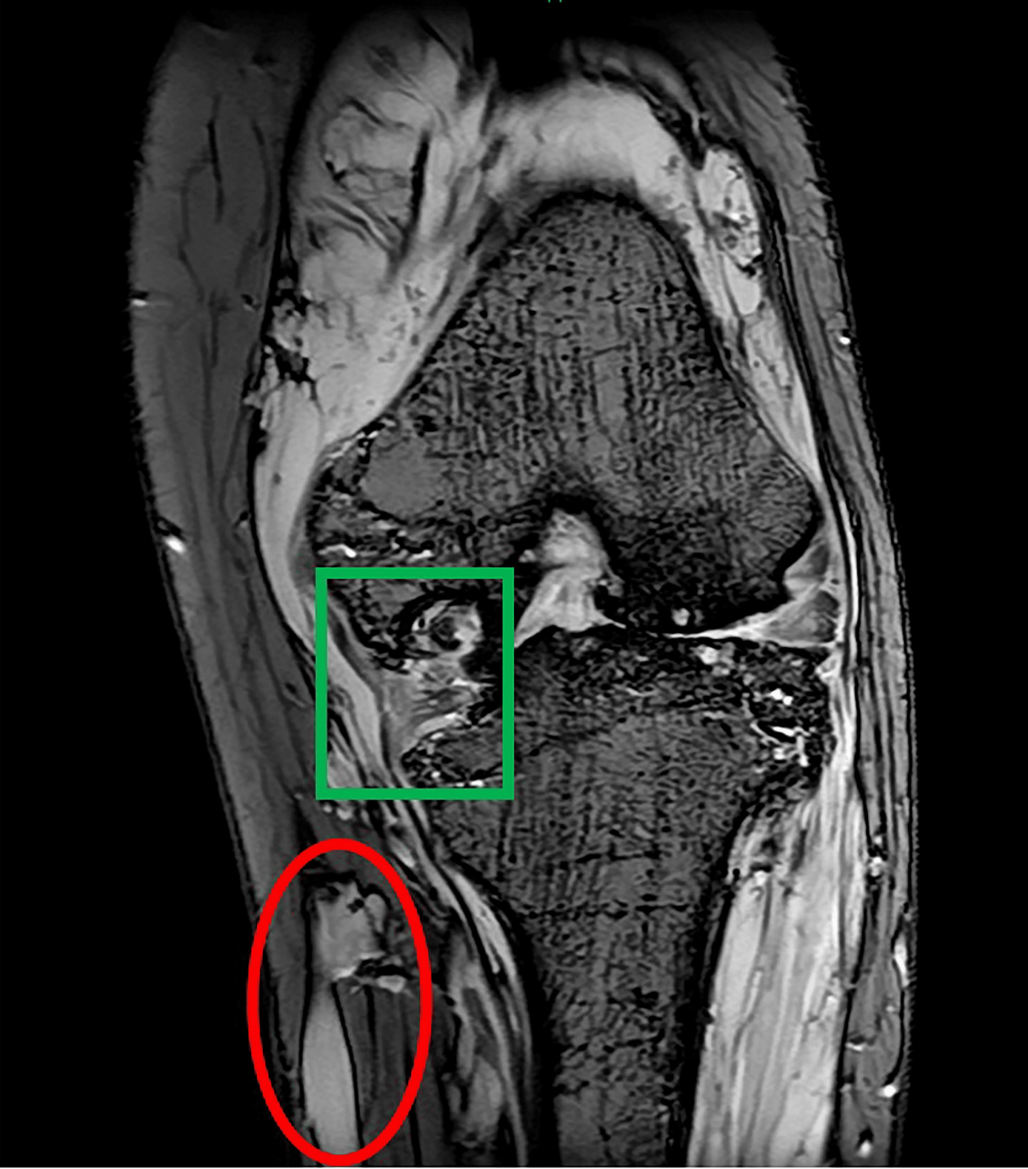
Preoperative T2*-weighted MRI findings. Enlarged veins and varicose veins (red circle) are visible, along with an arteriovenous malformation within the joint (green square). MRI, magnetic resonance imaging
